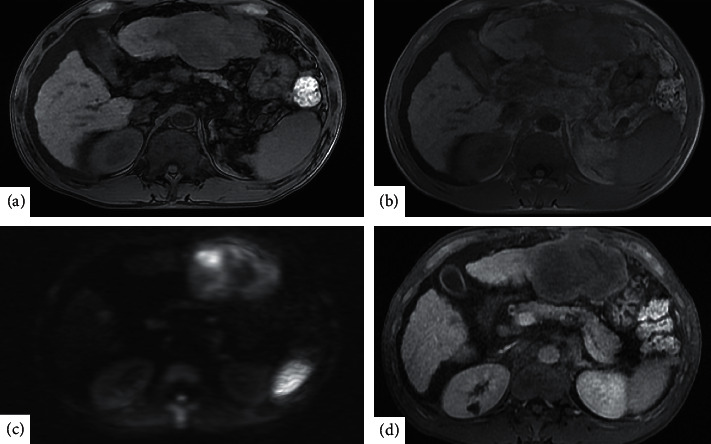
Marked hyperintensity on both the left and right hepatic lobe masses is revealed on diffusion-weighted magnetic resonance imaging (MRI), while peripheral arterial enhancement is seen on gadolinium ethoxybenzyl diethylenetriamine pentaacetic acid-enhanced MRI. (a) T1 in-phase. (b) T1 out-phase. (c) Diffusion-weighted image. (d) Hepatic phase.
